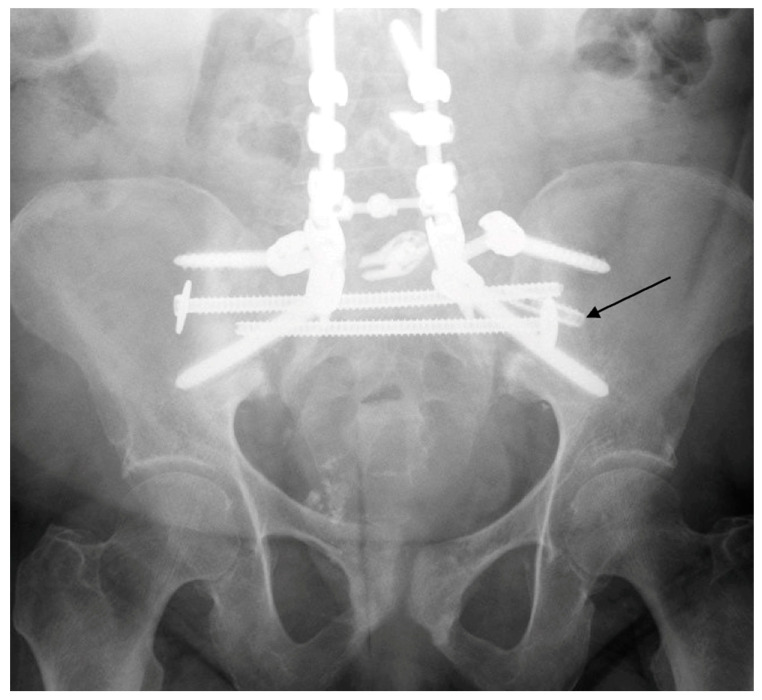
AP radiograph of revision spinopelvic fusion with retained broken screw from previous construct.
